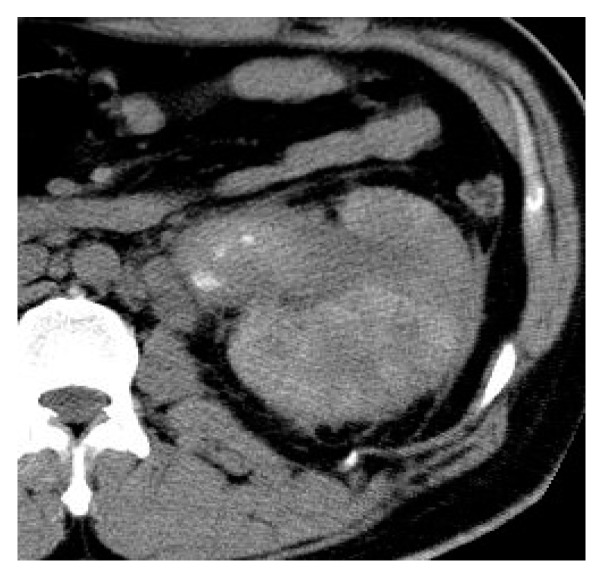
Left renal fungus infection. Axial non-contrast CT image shows hyperdense lesion at the renal pelvis with calcific foci inside.
